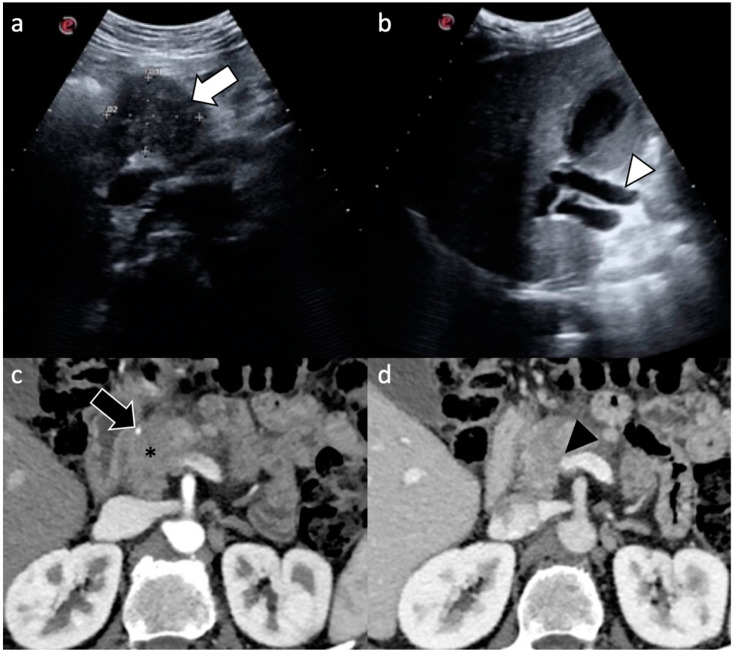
A 57-year-old man who came to the emergency department for jaundice and abdominal pain. (a,b) US detected a mass in the pancreatic head (white arrow) causing upstream dilatation of the common bile duct (white arrowhead); (c,d) Pancreatic CT scan confirmed the presence of mass in the pancreatic head (*) that caused encasement of the gastroduodenal artery (black arrow) as well as encasement and narrowing of the superior mesenteric-portal venous confluence (black arrowhead), the superior mesenteric vein, and the portal vein.
